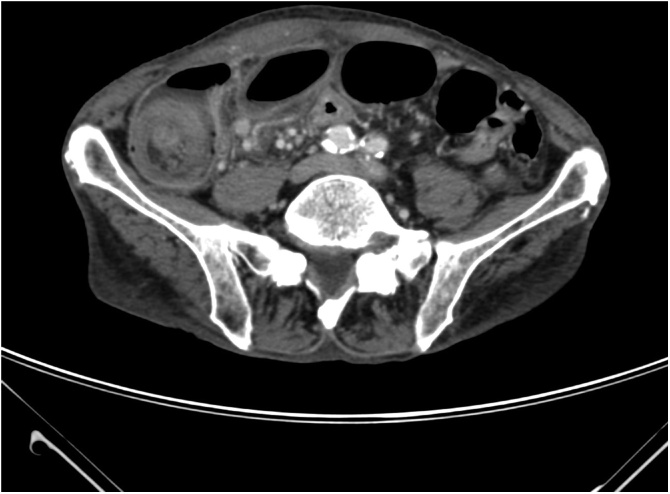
Classic “Bulls eye” appearance with concentric rings in a CT scan axial view; evidence of solid mass of the terminal ileum with stratified walls in the lumen.
